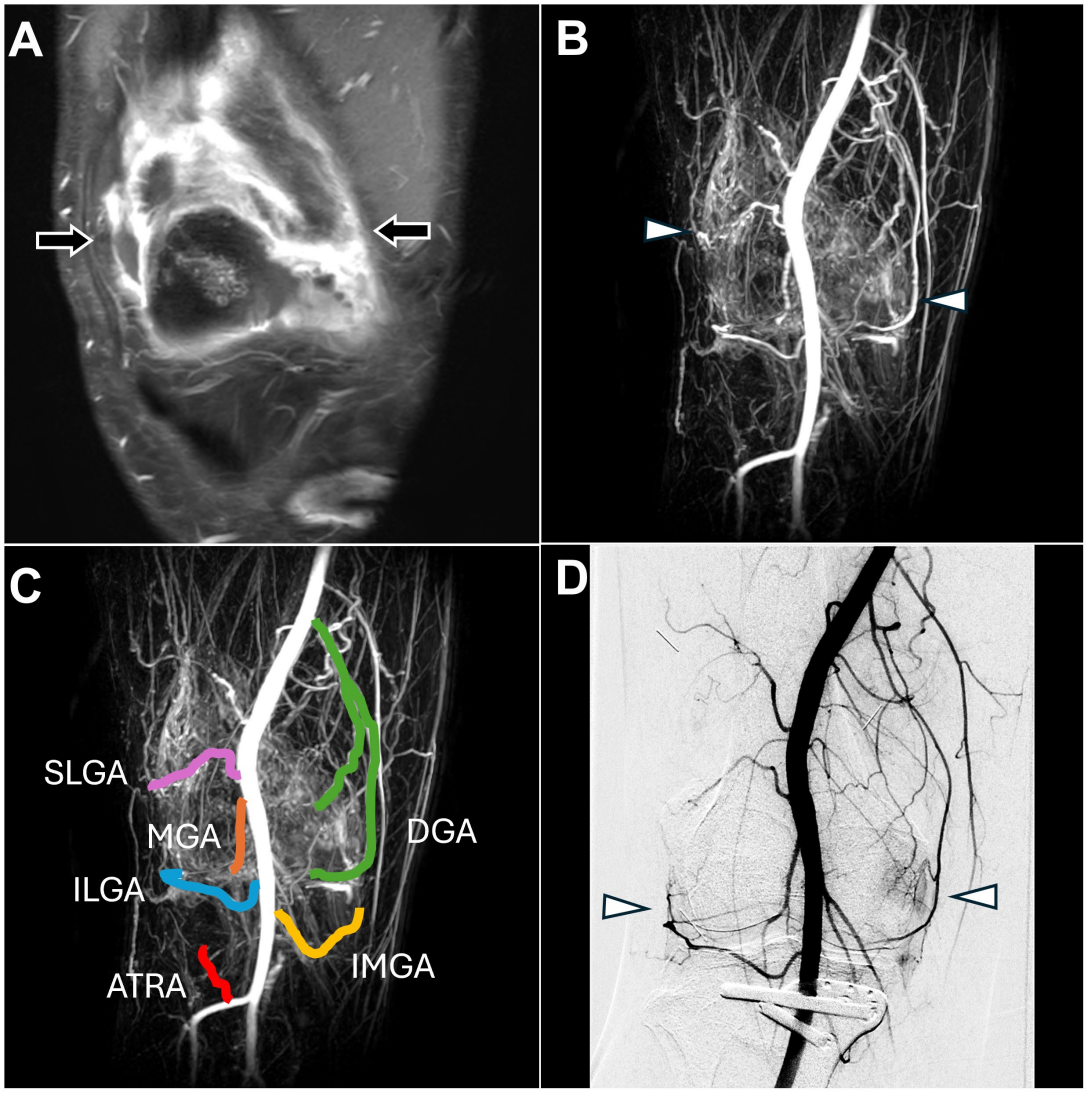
Dynamic contrast-enhanced magnetic resonance angiography and digital subtraction angiography images of refractory right knee pain after osteotomy. (A) Post-contrast fat-saturated T1-weighted images demonstrated synovitis with enhancement of the right knee synovium (black arrow). (B) DCE-MRA showed angiogenesis in the right knee synovium (white arrowhead), consistent with the DSA findings in (D) and enhanced MRI findings in (A). (C) DCE-MRA depicted all TAME-engaged vascular branches, including the SLGA, ILGA, MGA, ATRA, DGA, and IMGA. (D) The DSA via the popliteal artery showed angiogenesis surrounding the knee joint (white arrowhead). (DCE-MRA, dynamic contrast-enhanced magnetic resonance angiography; DSA, digital subtraction angiography; TAME, transarterial microembolization; SLGA, superior lateral genicular artery; ILGA, inferior lateral genicular artery; MGA, median genicular artery; ATRA, anterior tibial recurrent artery; DGA, descending genicular artery; IMGA, inferior medial genicular artery).
